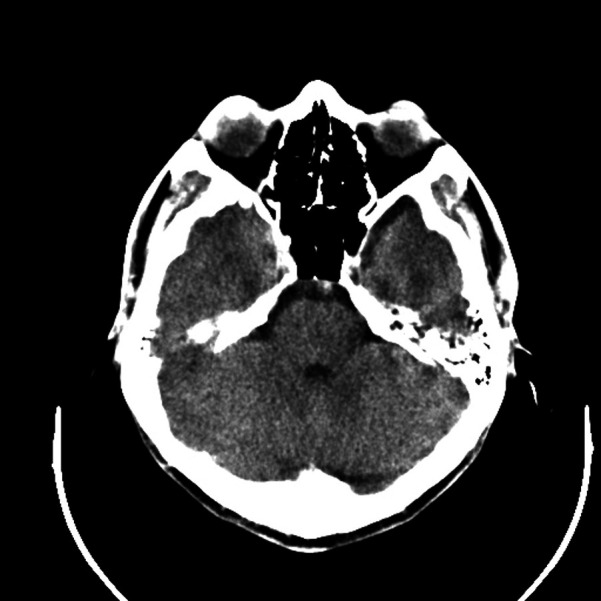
Head CT scan revealed a benign lesion located in the left temporal region.
